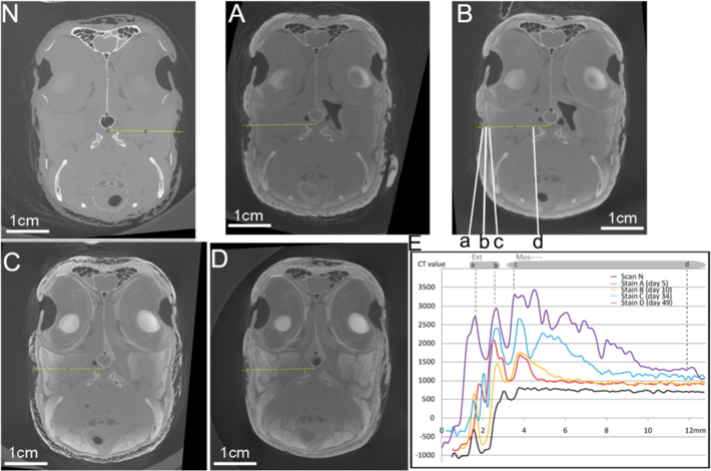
The coronal section of CT images crossing the eye and adductor chamber (n, a-d) with CT plots for the adductor region (e). n, a, b, c, and d correspond the respectively same scan as in Figs. 1 and 2; CT plots indicate an overall decreasing trend from the exterior margin of the muscle to more interior side (see detail in text). Exterior region and interior muscles were well identified and correlated with CT images and the plots (b, e: *a-d*). The same color regime was used for the CT plots as in Figs. 1 and 2. Anatomical abbreviations: Ext, external zone; Mus, muscles
